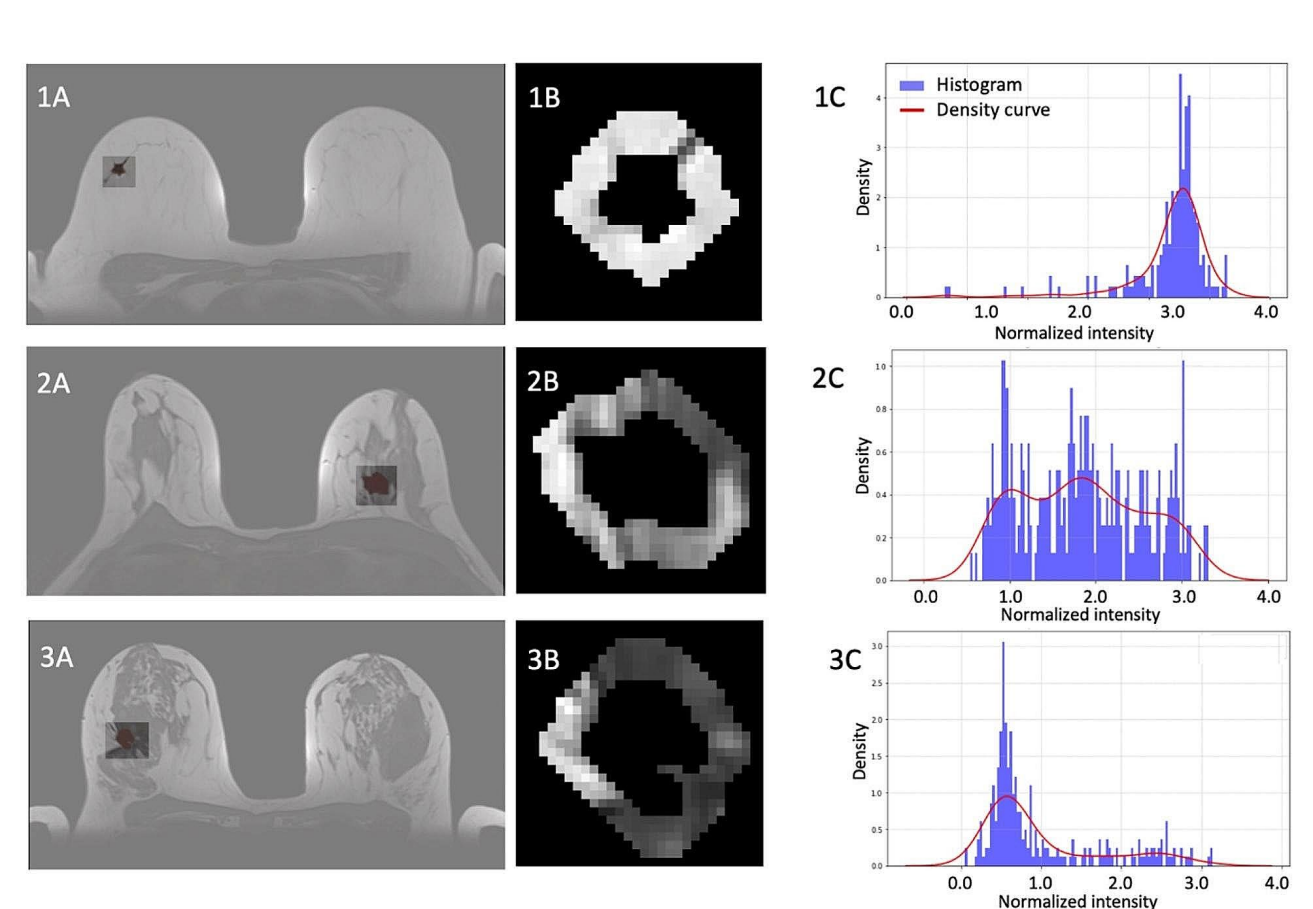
Quantitative analysis of the peri-tumor environment (pTE) in T1-weighted (T1w) magnetic resonance imaging. The panels display: (A) original T1w sequence with tumor segmentation overlaid for three cases labelled 1, 2 and 3, (B) corresponding segmentation of the 5 mm-thick outer margin, depicting the peritumoral region; and (C) a histogram representing the intensity distribution from the peritumoral region, enhanced with a superimposed density curve, for 3 patients with mostly fatty pTE (1), mixed fibroglandular and fatty pTE (2) and mostly fibroglandular pTE (3). The shape of the density curve and the position of the peaks vary in these 3 patients, depending on the relative proportion of fat (high intensity pixels) and fibroglandular tissue (low intensity pixels). pTE: peri-tumor environment, T1w: T1-weighted MRI
